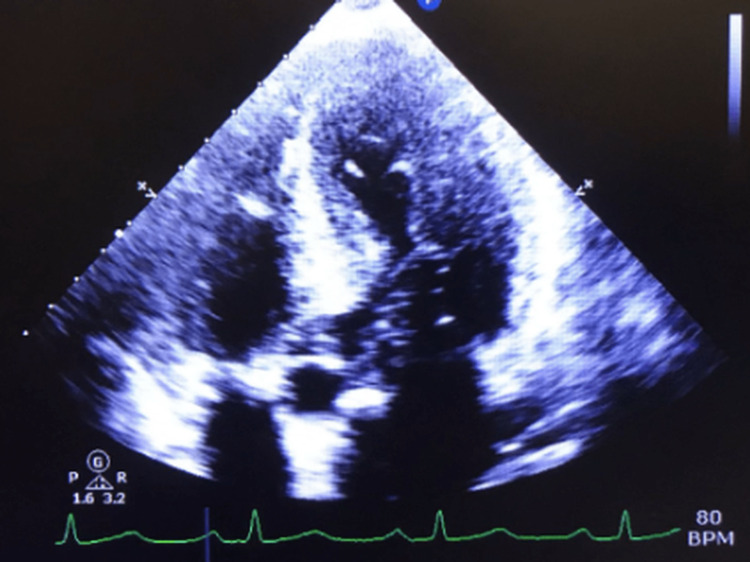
Cardiac transthoracic echocardiogram (TTE) showing infiltrative cardiomyopathy without decreased ejection fraction.
